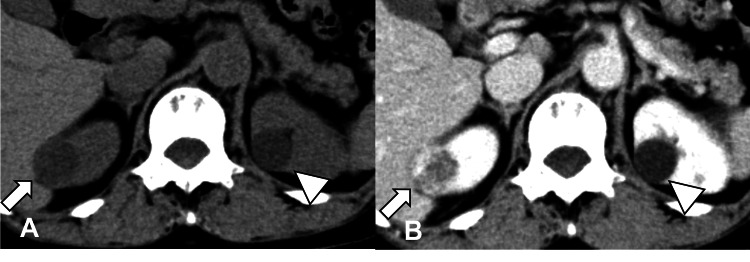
CT images of a 39-year-old man with clear cell RCC A: unenhanced image; B: enhanced image in nephrographic phase
